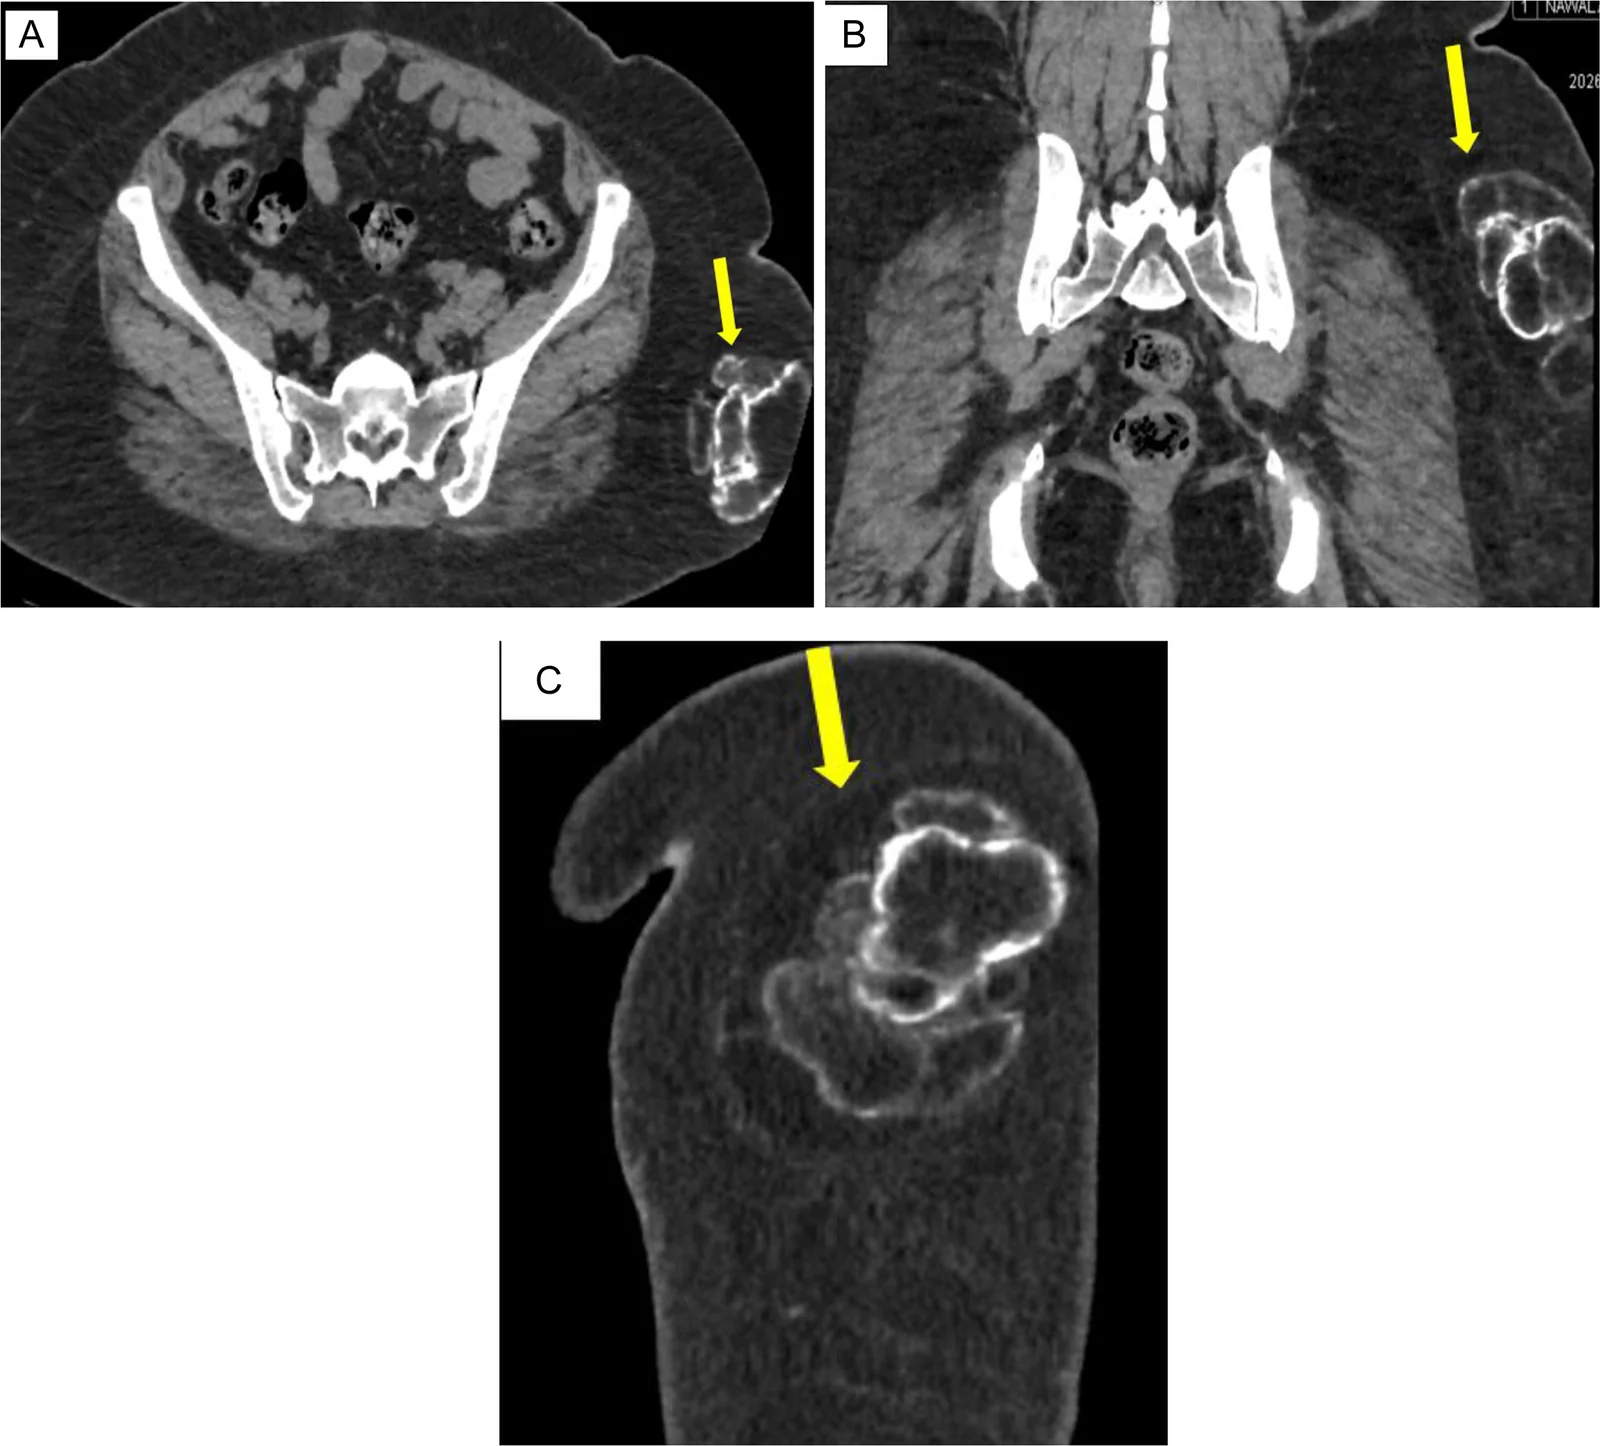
CT scan of the pelvis in soft-tissue window, axial (A), coronal (B), and sagittal (C) planes, showing a lobulated, well-circumscribed mass of fat density with internal calcifications, located within the subcutaneous soft tissues of the left gluteal region (yellow arrow).
